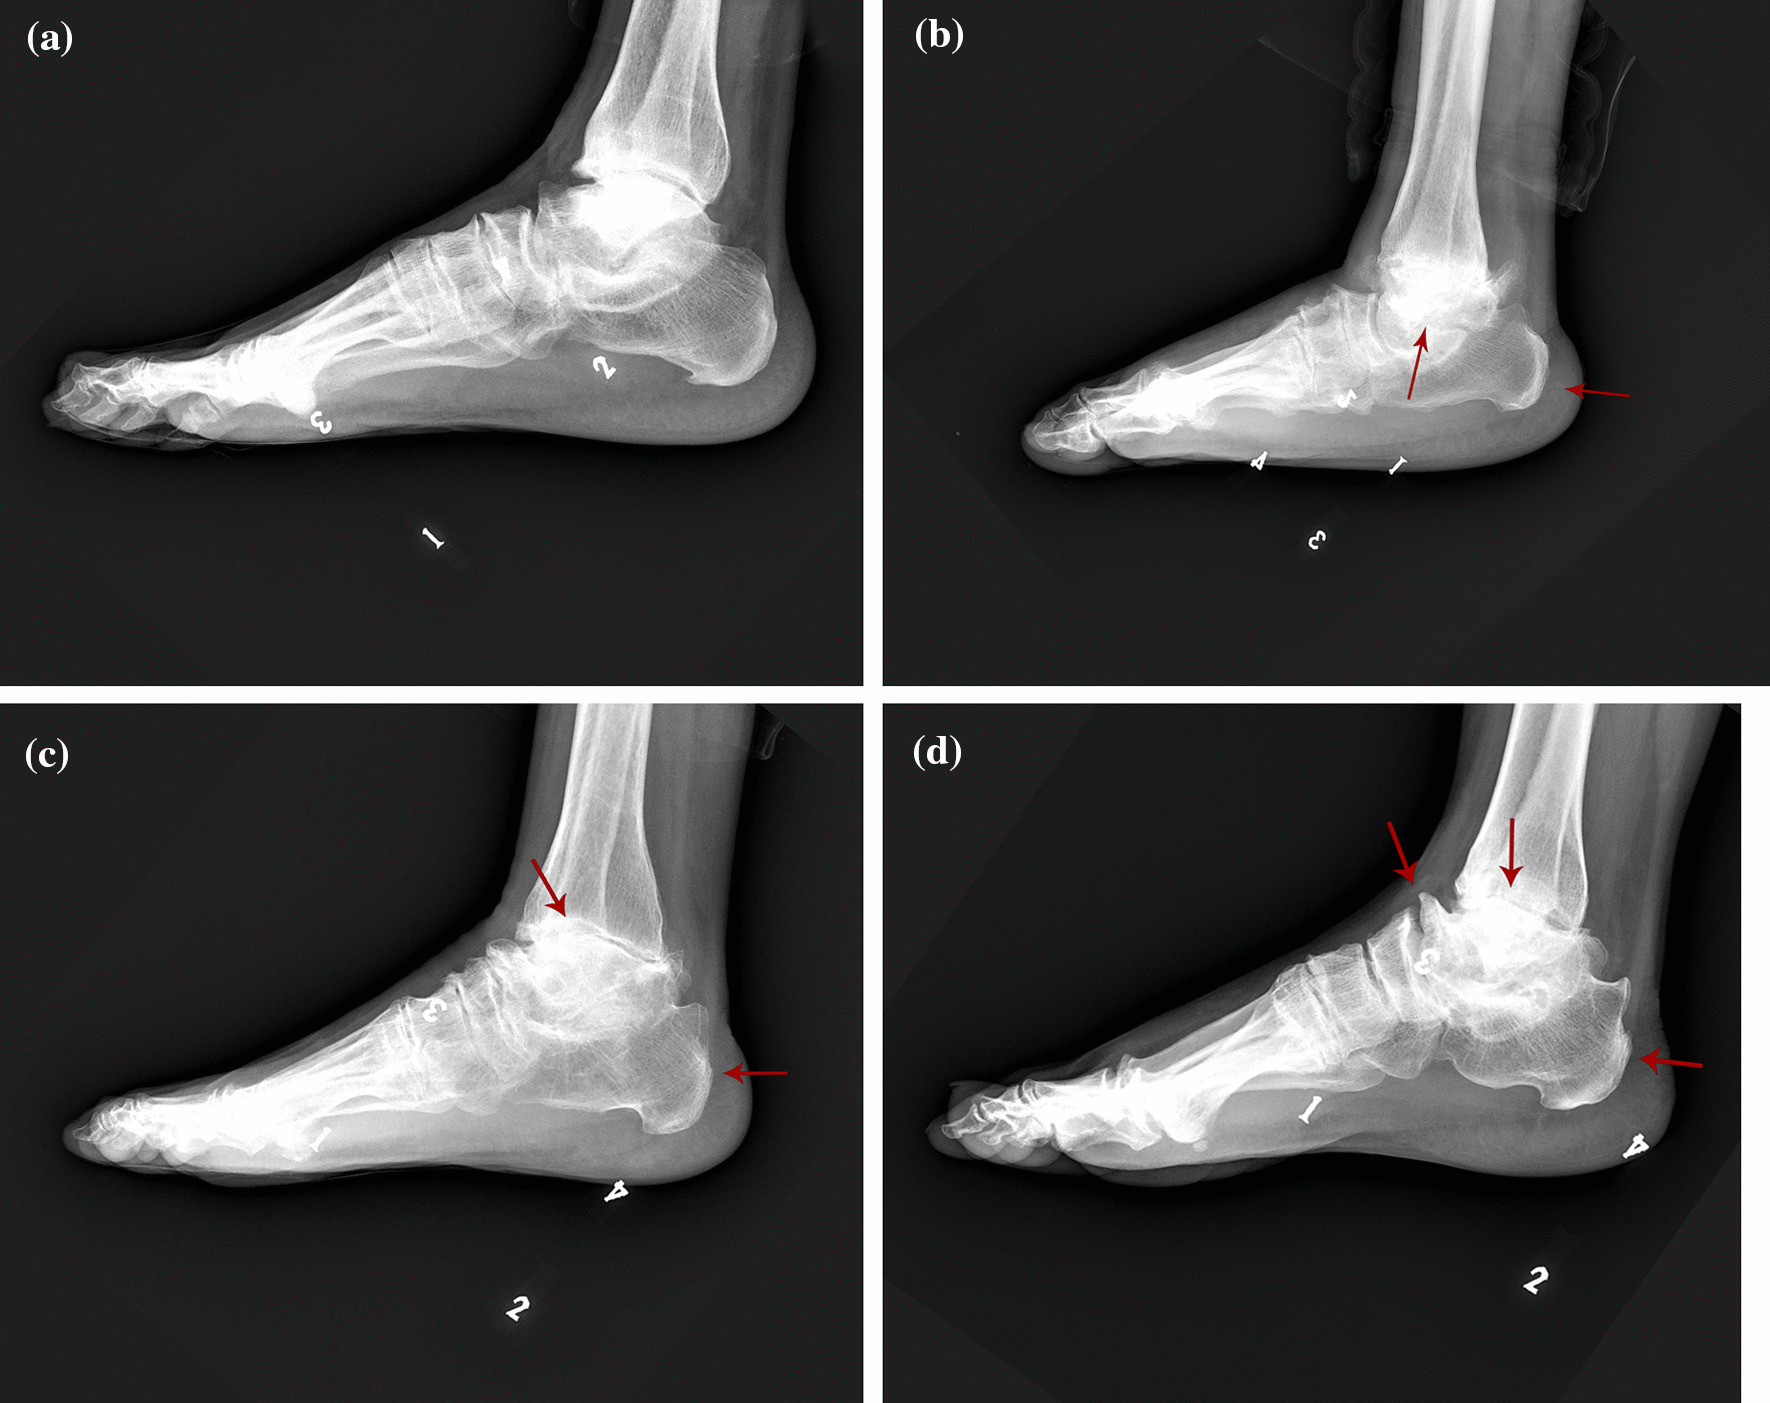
X-ray images of lateral ankle joint in patients with KBD and normal controls. aNormal adult ankle joint X-ray image: normal calcaneus length, no hyperplasia of the talar head and body, and smooth articular surface of the talus. b X-ray image of degree I ankle joint changes: the calcaneus is slightly shorter, the talar head and body are hyperplasia, and the articular surface of the talus is smooth. c X-ray image of degree II ankle joint changes: the calcaneus is slightly shorter, the posterior and superior border of the calcaneus is hyperplasia, the head and body of the talus are hyperplasia, and the articular surface of the talus is rough and uneven. d X-ray image of degree III ankle joint changes: short calcaneus, hyperplasia of talus head and talus body, upturned talus head and navicular bone to form bone spurs and peripheral cuneiform spurs, talus articular surface collapsed and flattened
